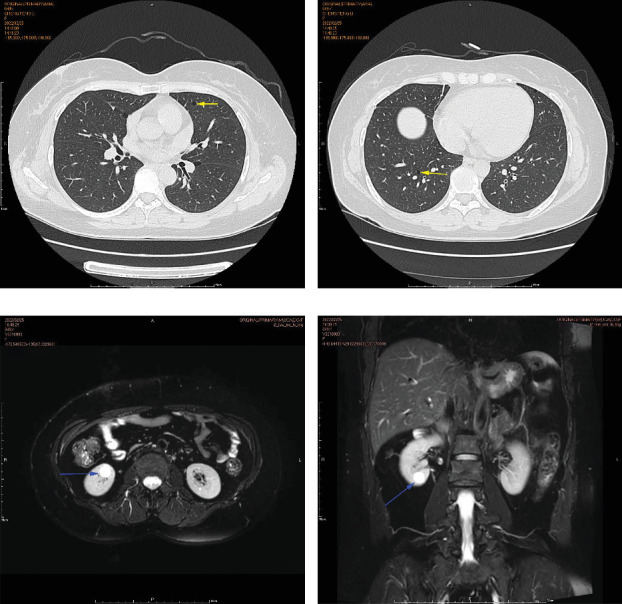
Chest CT and renal MRI results of proband's Sister 1. (a, b) Multiple diffuse cystic changes in both lungs. Chest CT showed multiple diffuse cystic changes in both lungs, thin wall, irregular shape, and uneven size, located in both lower lungs and mediastinum. Yellow arrows indicate lung cystic. (c, d) Renal magnetic resonance plain scan and dynamic enhancement of the left renal cyst indicated a long T2 signal shadow with a clear punctate boundary in the left kidney (c) and the lesion signal did not decrease in the reverse phase image (d). No abnormal enhancement was found in the dynamic enhanced scan. Blue arrows indicate renal cyst.
